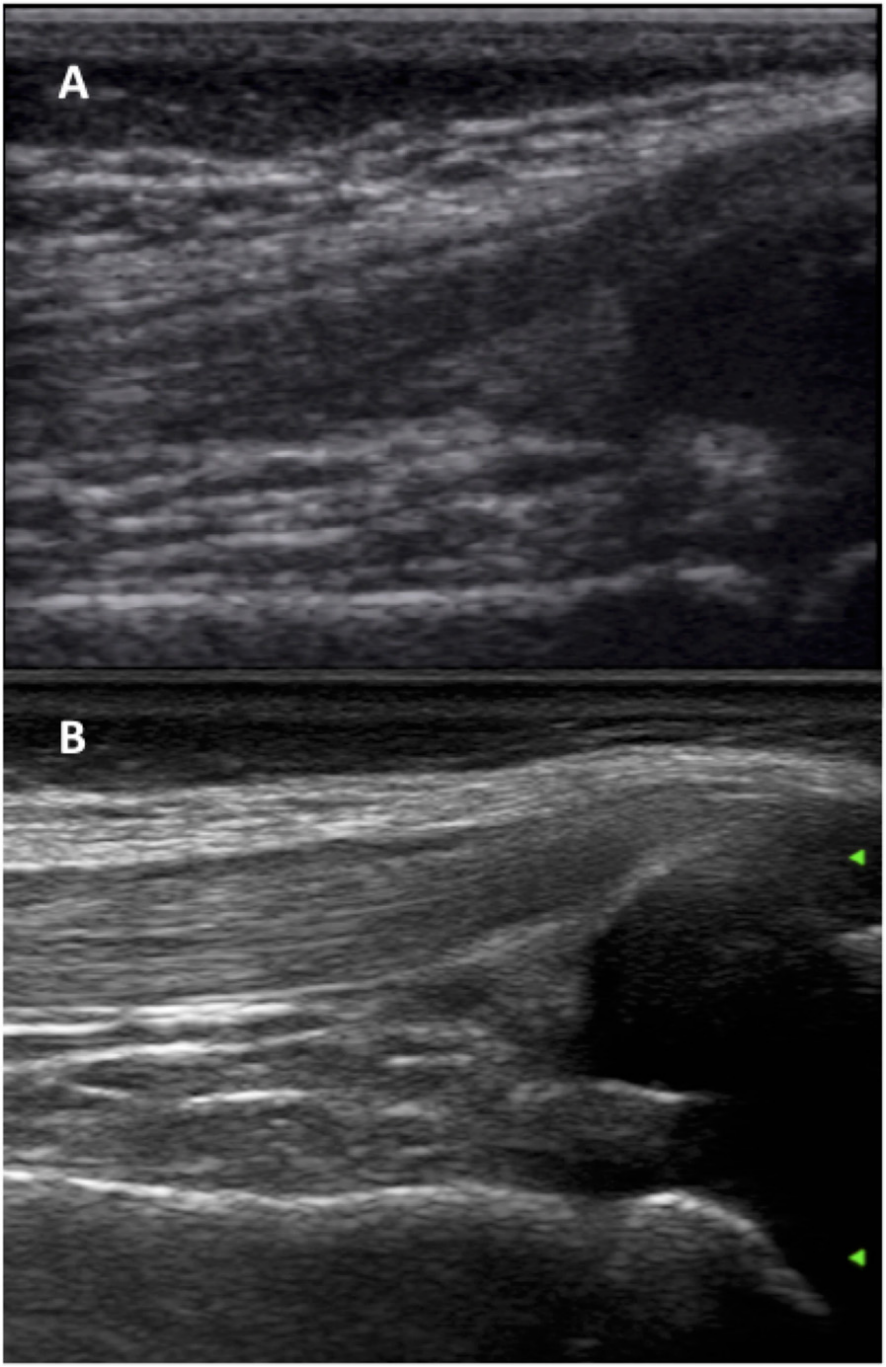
Longitudinal scan of the suprapatellar recess in two 7 years old boys performed with high multifrequency probe: a. Top-level ultrasound machine in the early 2000’s; b. Top-level ultrasound machine in 2015
